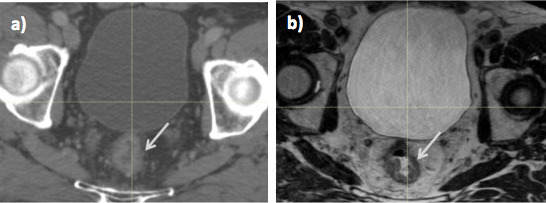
Radiotherapy planning imaging in a male patient with T3N1 rectal cancer; (a) CT and (b) MRI. On MRI, the tumour (arrow) is easily differentiated from normal rectum, which is not possible on CT.
